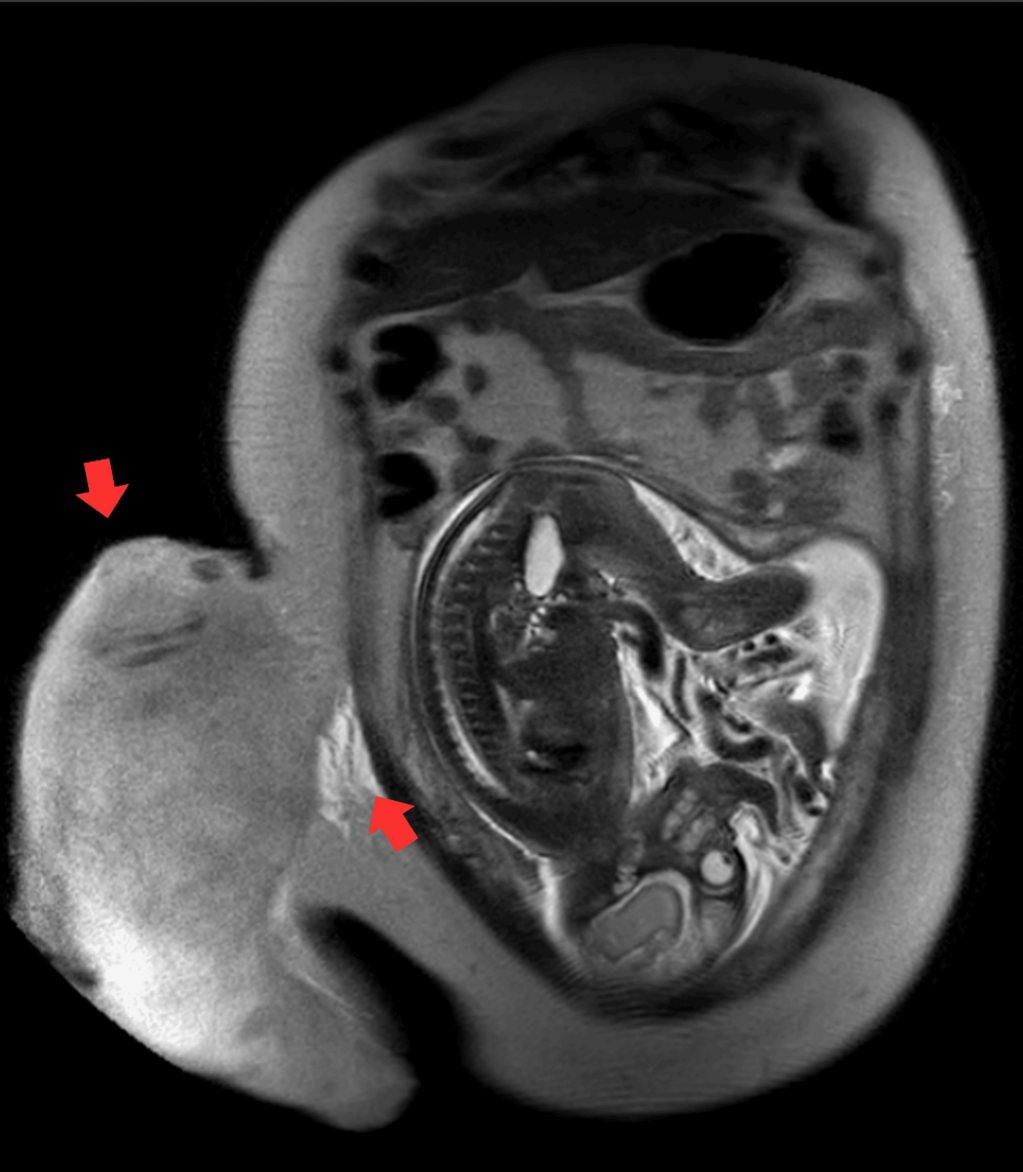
Sagittal MRI view shows a large muscle-derived tumor measuring 25 × 18 × 21 cm. MRI: Magnetic resonance imaging
